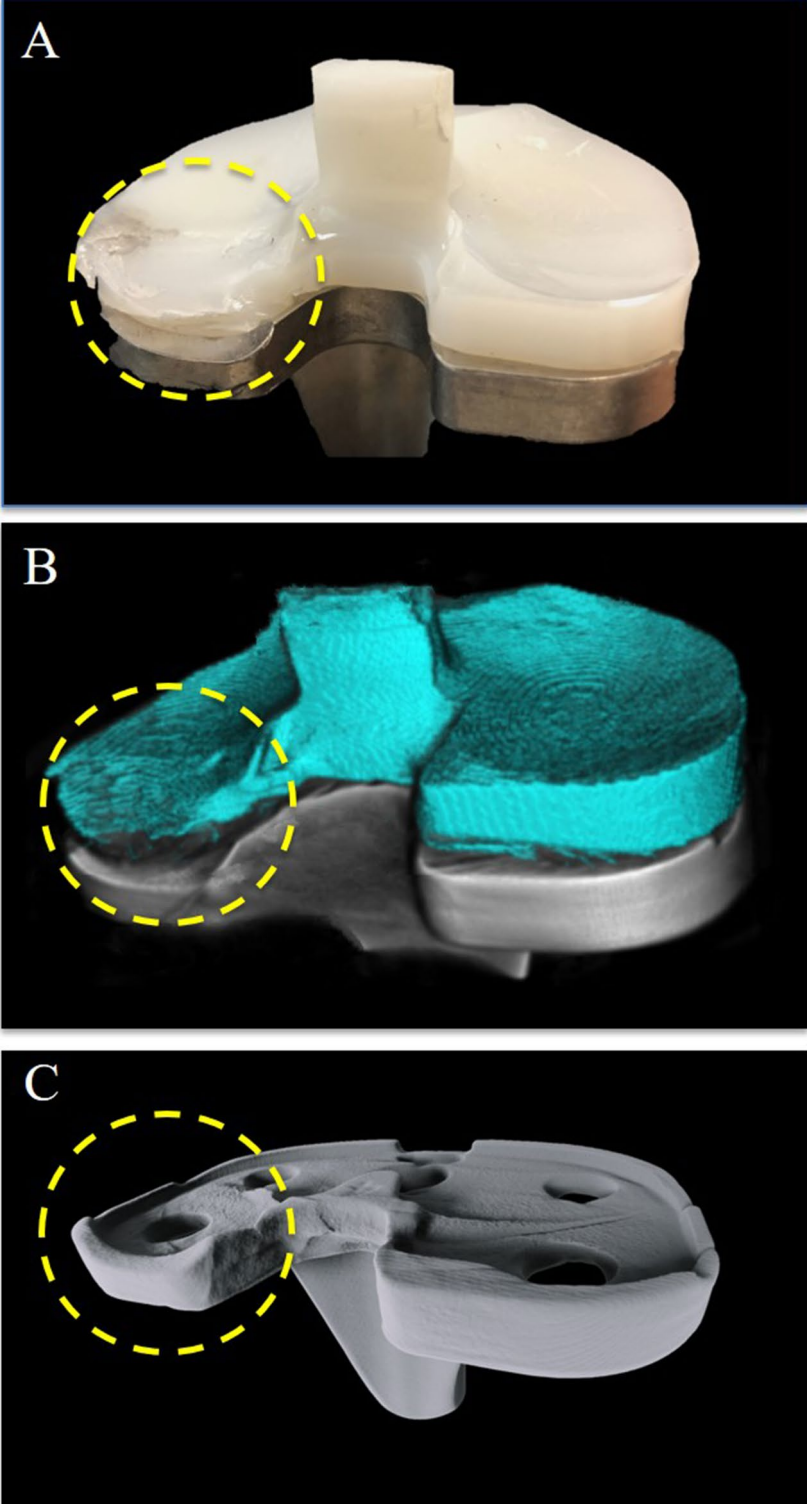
(a) Clinical photos, (b) MARS-CT images and (c) MARS-CT images with polyethelene digitally removed. The wear at the postomedial aspect of the polyethylene impant and tibial metallic tray is clearly visible (circle).
